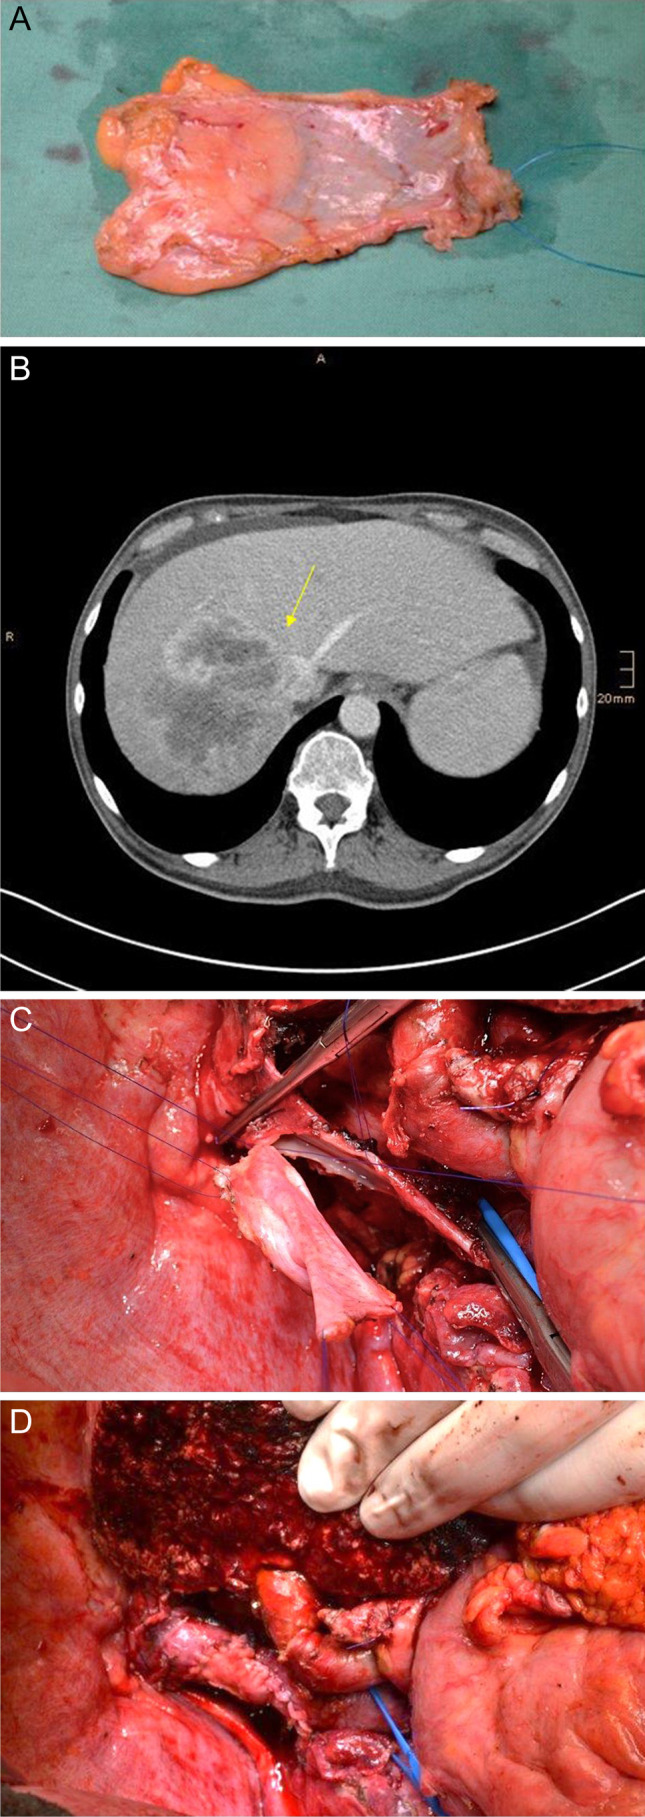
Patient with iCC of the right liver lobe infiltrating the right and middle hepatic vein as well as the IVC. Vena cava reconstruction was performed with peritoneal patch after tangential resection of the vena cava and resection of the middle hepatic vein combined with extended right hemihepatectomy. a Patch harvested from the parietal peritoneum. b Patient’s CT scan shows the tumor (arrow) infiltrating the IVC, the right and middle hepatic vein. c and d Reconstruction of vena cava after tangential resection by using the peritoneal patch
